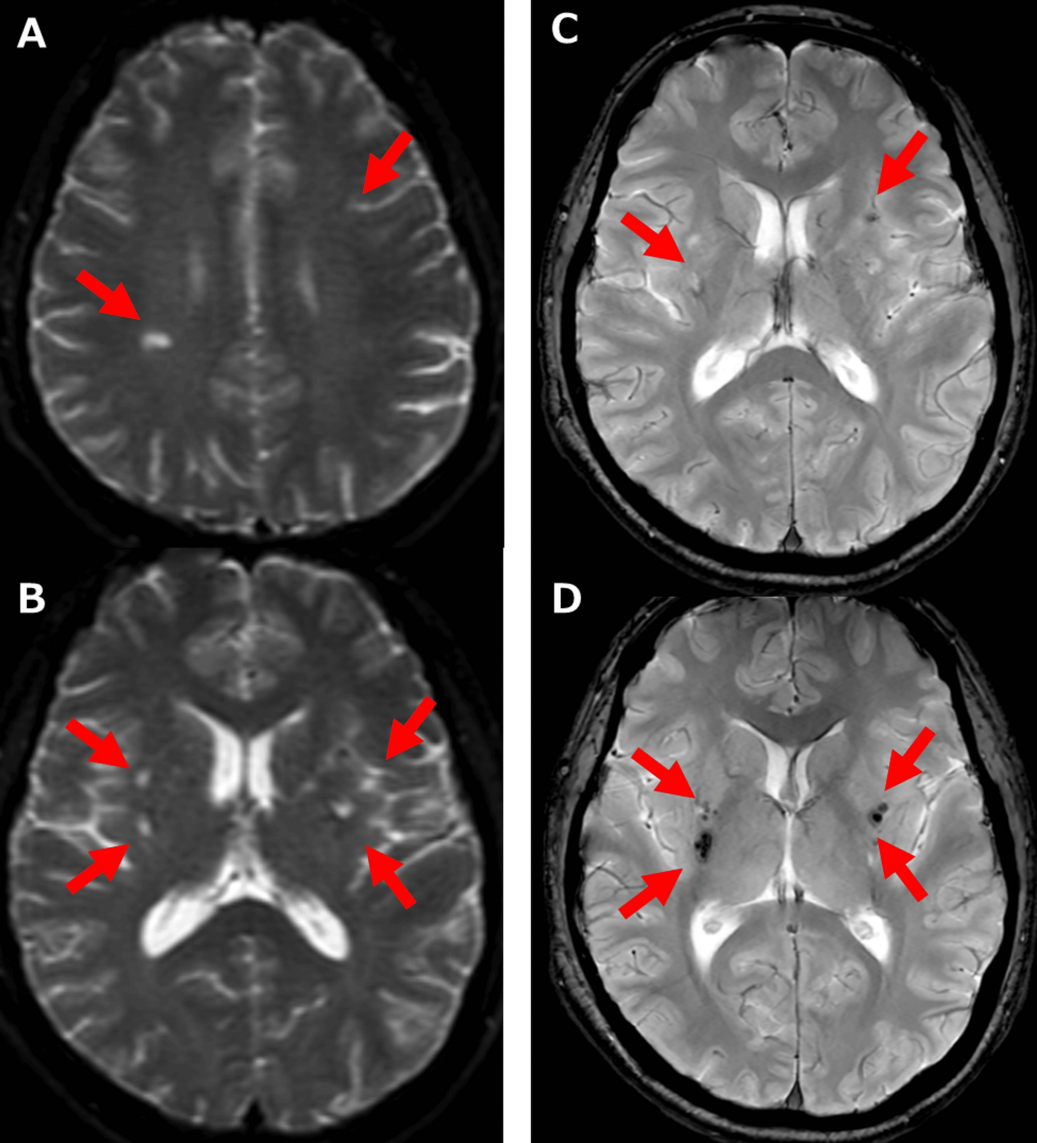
Brain MRI A, B: Diffusion-weighted images showing multiple increased signal intensities (arrows), indicating multiple cerebral infarctions. C, D: T2 star-weighted images revealing multiple decreased-signal intensities in the basal ganglia (arrows), demonstrating multiple microbleeds. MRI: magnetic resonance imaging
